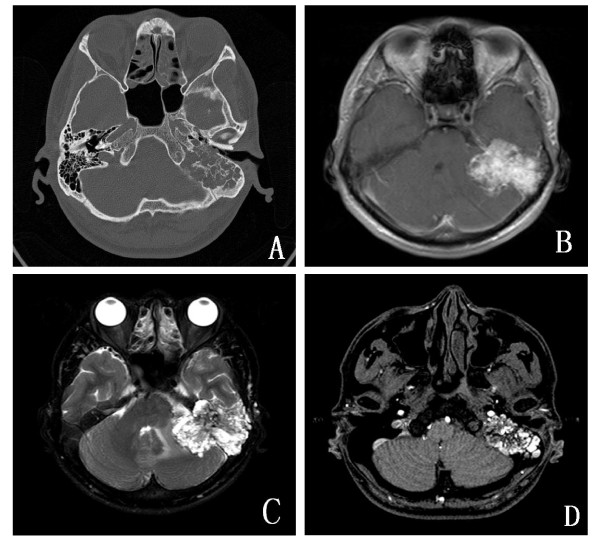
Radiologic characterization of endolymphatic sac tumor. A. CT imaging demonstrated an expansile lytic lesion of the mastoid process of the left petrous bone extending to involve both the left medial mastoid as well as the middle ear. B. MR scan showed a 5.2 cm × 4.7 cm × 4.2 cm mass which was irregular, heterogeneous, and lobulated and showed hyperintensity on T1-weighted images. C. The periphery of the lesion appeared markedly hyperintense on the T2-weighted images. D. Axial postcontrast T1-weighted MR scan showed a heterogeneous enhancing mass.
